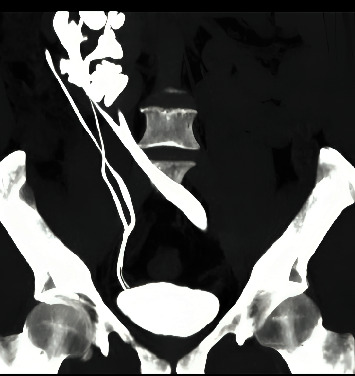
CT urography showed that the right kidney had a complete duplex system with two separate ureters, while an incomplete duplex system was found on the left side.
